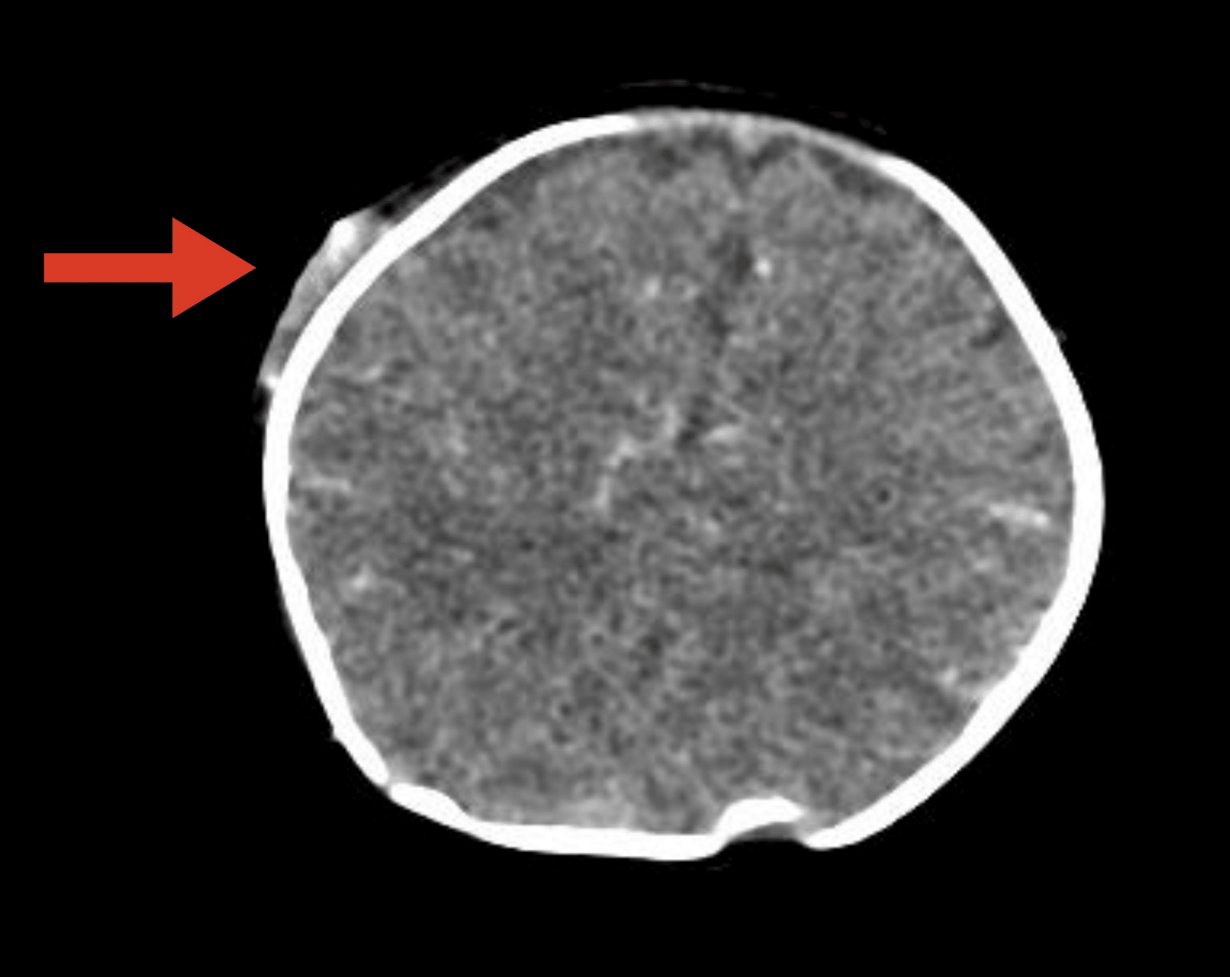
Contrast-enhanced computed tomography showed the mass with rich blood flow component.
